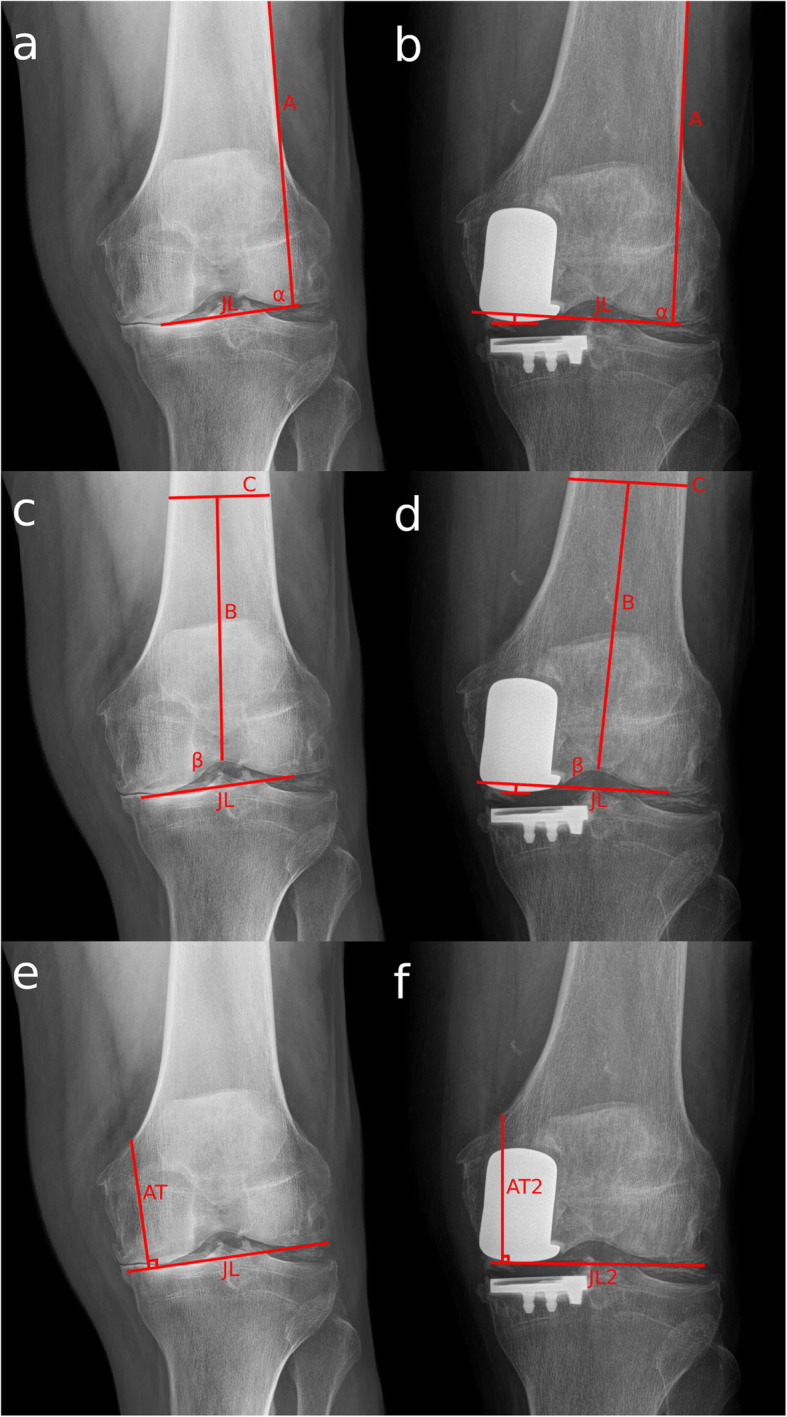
Anteroposterior Knee X-rays of a left knee showing the different joint line measure techniques. In the Weber 1 method, the angle (alpha) between a line that goes through the most distal point of both femoral condyles (JL) and the lateral femoral cortex (a) is obtained in the pre-operative x ray (a). Then (alpha) is reproduced in the post-operative x ray (b) with the lateral femoral cortex and the non-operative femoral condyle as reference. The variation in joint line is then measured. In the Weber 2 method the angle (beta) is obtained in the pre-operative x ray (c) between a line that goes through the most distal point of both femoral condyles (JL) and the line B that goes from the most proximal point of the intercondylar notch and the center of a line 10 cm proximal to it (c). Then the angle is reproduced in the post-operative x ray (d) with line B and the non-operative condyle as reference. The variation in joint line is then measured. In the Iacono method, the distance (AT) between the most proximal point of the Adductor Tubercle perpendicular to a line that goes through the most distal point of both femoral condyles (JL) (e) is compared to the same distance in the post-operative x ray (f). The difference between both is the variation in joint line
